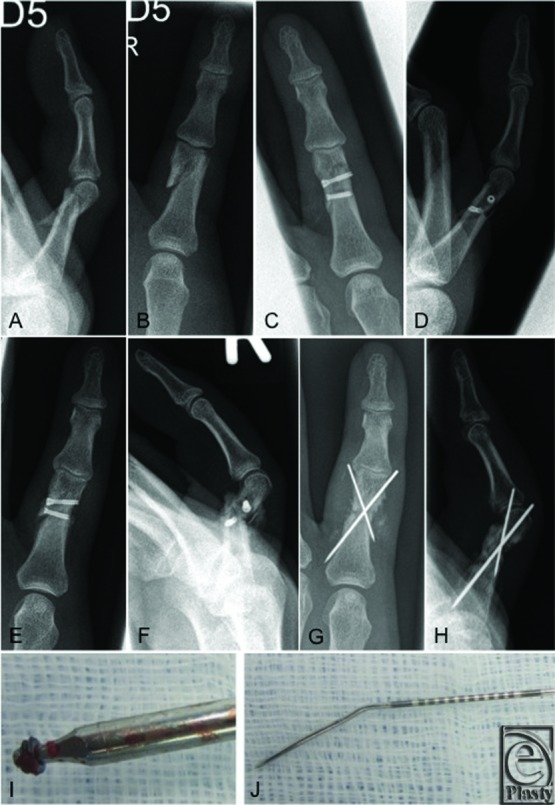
Presentation of a case. A 43-year-old patient undergoing a dislocated spiral fracture of the proximal phalanx of digiti minimi (a, b). The postoperative radiographs after the screw osteosynthesis (c, d). Note the missing head of 1 screw (c). Two weeks after the surgery, a secondary displacement of the fracture appeared (e, f) and was corrected by a new K-wire osteosynthesis (g, h). The broken screwhead (i) and the bent 0.7-mm K-wire used for the screw osteosynthesis (j).
